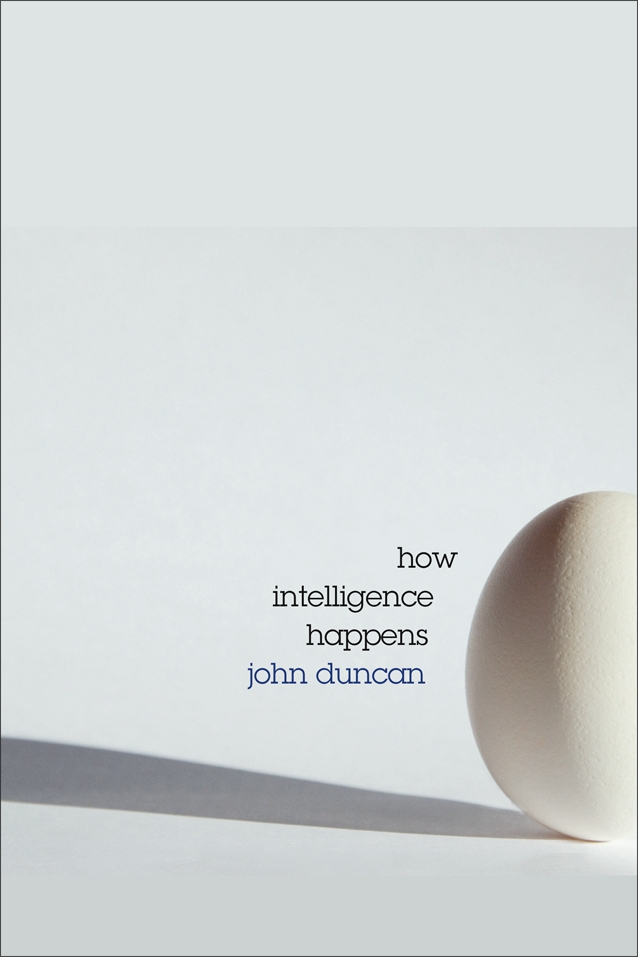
Duncan J (2010) How Intelligence Happens. New Haven, CT: Yale University
